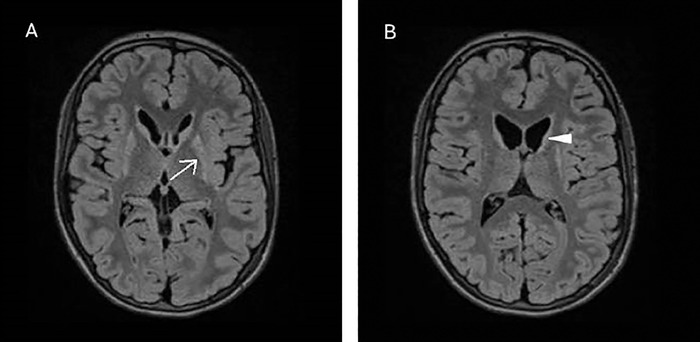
3D SPACE (sampling perfection with application-optimized contrasts using different flip angle evolutions) FLAIR (fluid-attenuated inversion recovery) magnetic resonance images of the brain of Case 1 show atrophy of the bilateral caudate heads and putamina, with associated signal increase in the putamina. The left putamen is identified with a white arrow in image A. The left caudate head is identified with a white arrowhead in image B.
